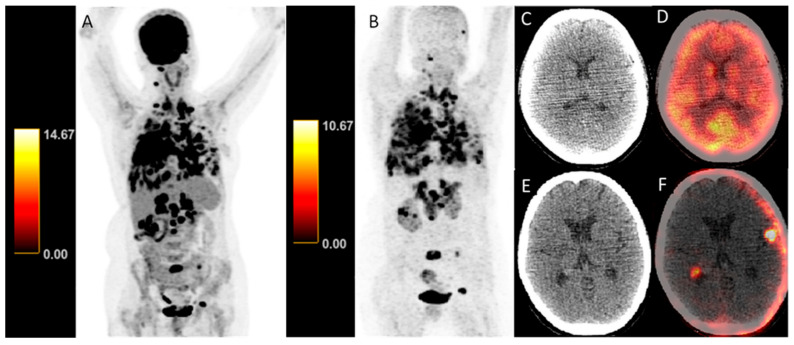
Maximum-intensity projection (MIP) and axial PET/CT images demonstrate differential tracer uptake in a patient with metastatic lung cancer. Panels A and B show whole-body MIP images for [18F]FDG (A) and [68Ga]Ga-DOTA.SA.FAPi (B), highlighting primary tumor and multiple metastatic lesions in lungs, lymph nodes, and bones. Axial CT (C,E) and fused PET/CT (D,F) images depict intracranial metastases: c and d correspond to [18F]FDG CT and fused PET/CT, whereas e and f correspond to [68Ga]Ga-DOTA.SA.FAPi CT and fused PET/CT, demonstrating higher lesion-to-background contrast with [68Ga]Ga-DOTA.SA.FAPi SUV color bars displayed adjacent to the MIP images indicate the SUV scale used for PET image display and apply to both the MIP and corresponding axial fused images (0–14.67 for [18F]FDG and 0–10.67 for [68Ga]Ga-DOTA.SA.FAPi). Abbreviations: MIP, maximum-intensity projection; PET, positron emission tomography; CT, computed tomography.
